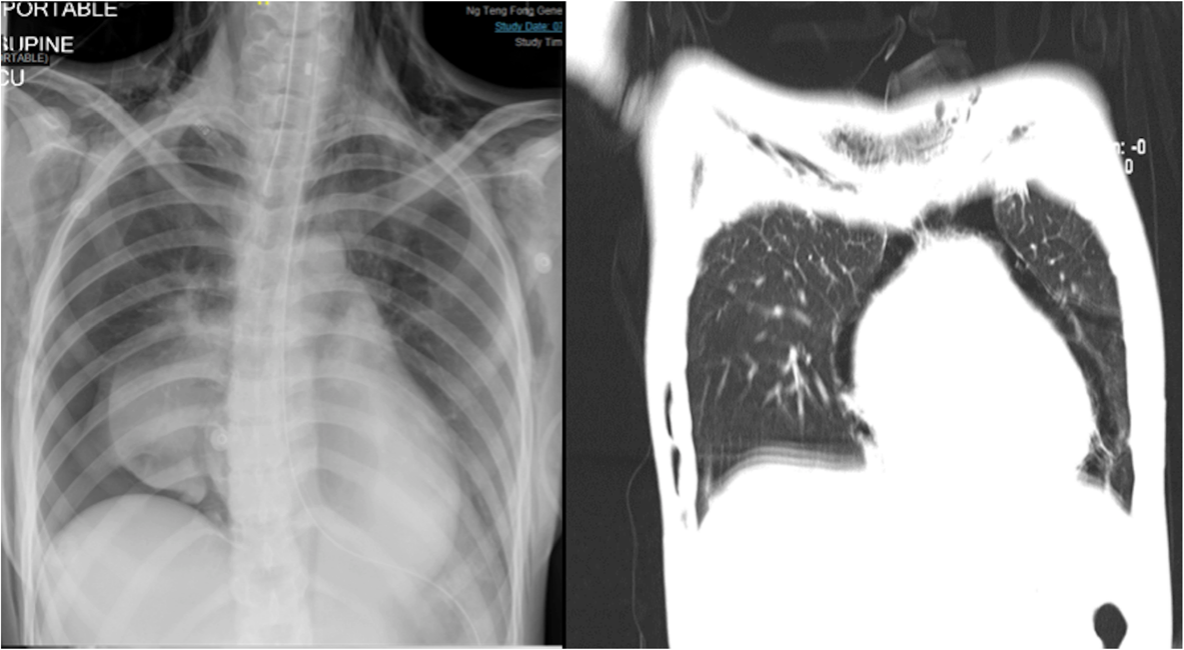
Chest radiograph showing subcutaneous emphysema, pneumothoraces, and pneumomediastinum (left); CT thorax demonstrating pneumomediastinum (right)
